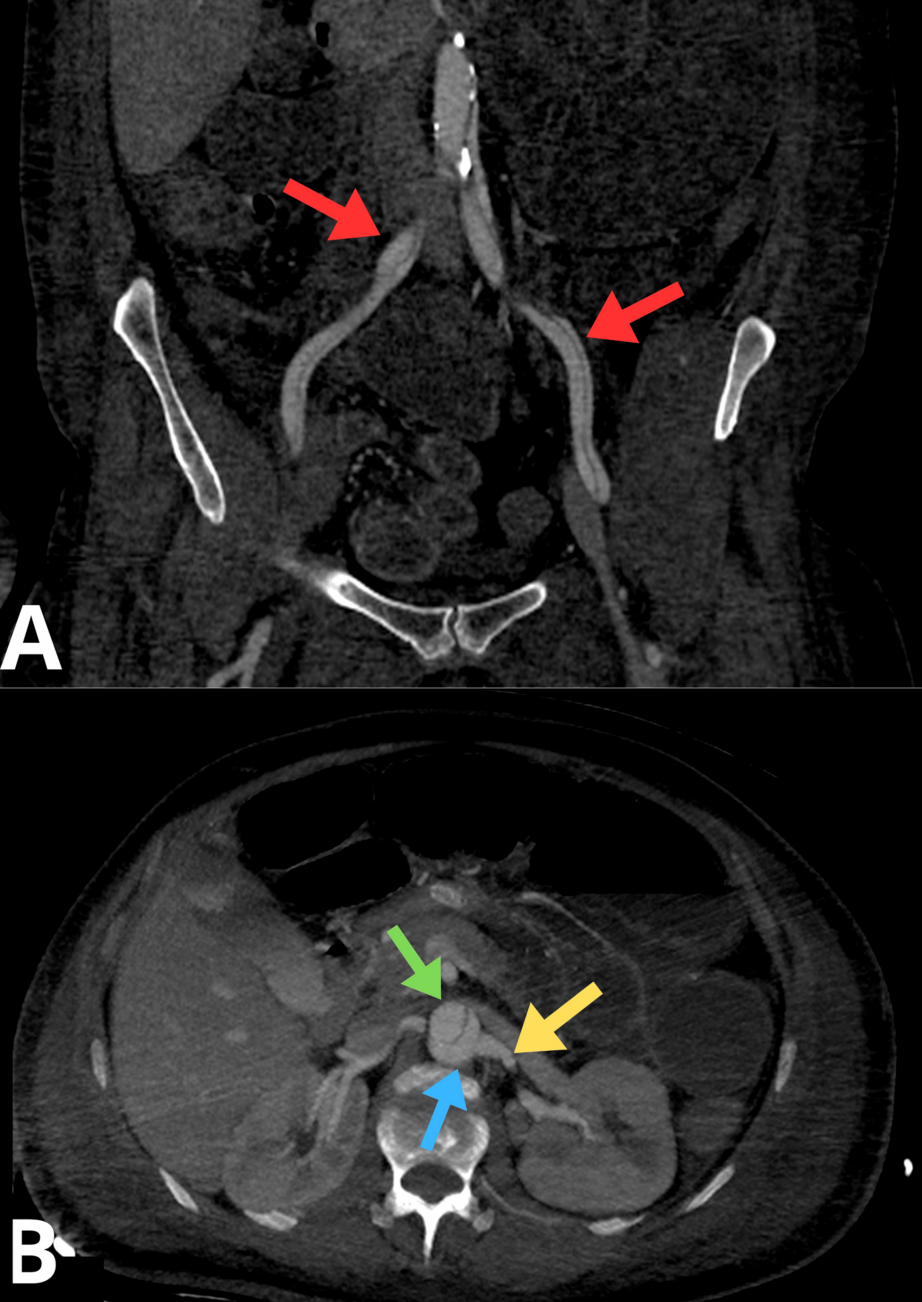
Angio-CT Scans: (A) Coronal Plane and (B) Transverse Plane The red arrow indicates dissection in both common iliac arteries. The green arrow indicates the true lumen. The blue arrow indicates the false lumen. The yellow arrow indicates the left renal artery originating from the false lumen CT: computed tomography
